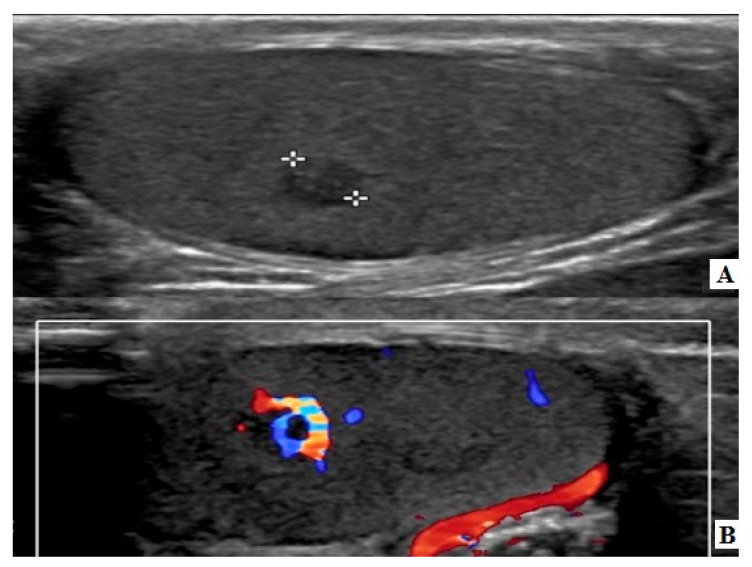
Ultrasound (US) images, in B (A) and color Doppler mode (B), depicting a hypoechoic lesion with intense vascularization due to a prominent peripheral arterial vessel.
